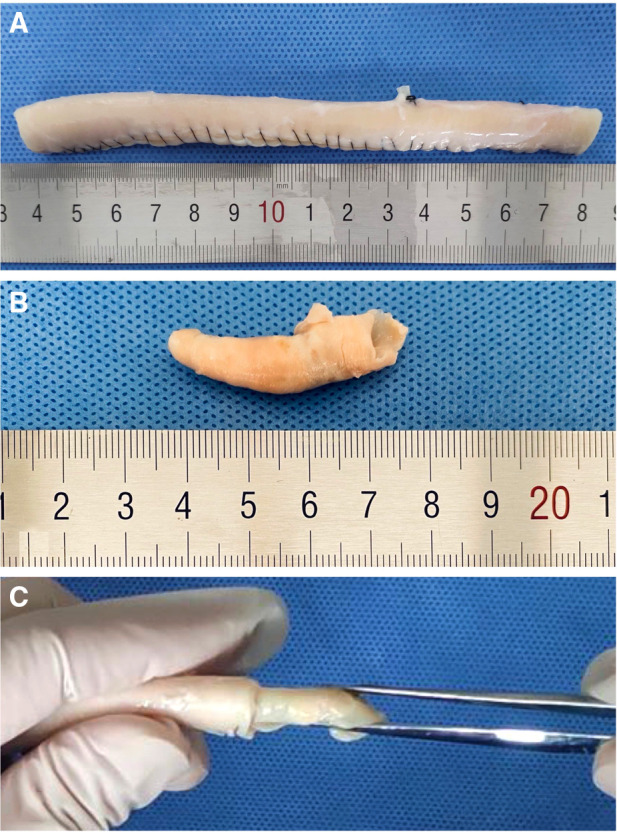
(A) Carotid artery made with a pruned and sutured descending aorta. (B) Atherosclerotic carotid artery plaque from the patient. It was confirmed as a stable plaque by ultrasonography. (C) We inserted the plaque into the “carotid artery” to make an atherosclerotic carotid artery occlusion state.
